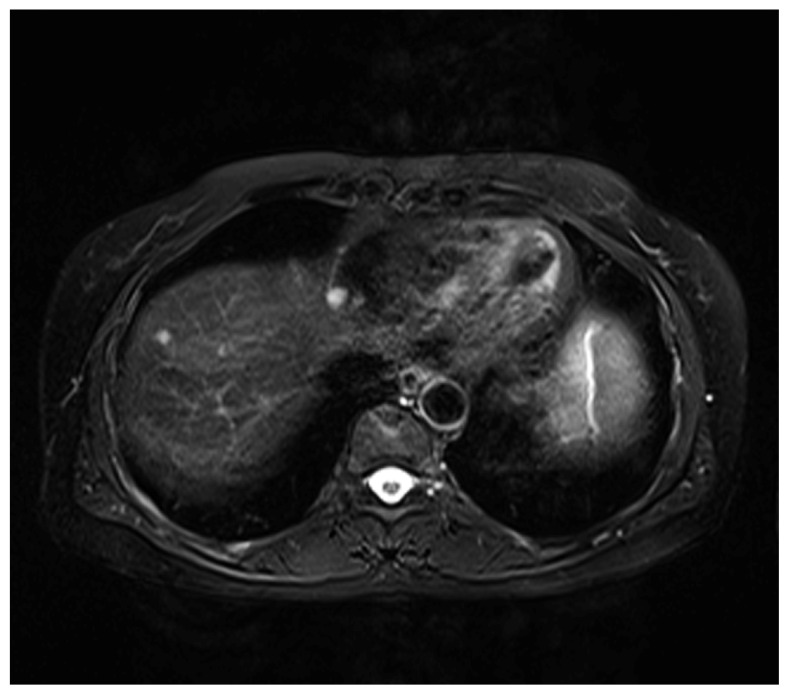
After receiving two courses of caspofungin acetate first-line therapy, follow-up horizontal abdominal MRI image showed evident remission.
